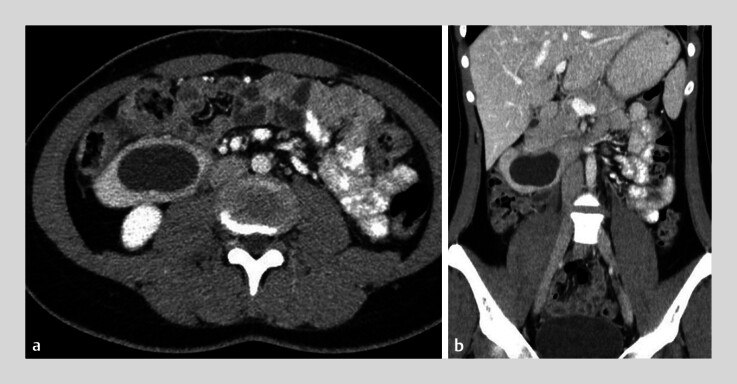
Computed tomography scan of the duodenal duplication cyst.
